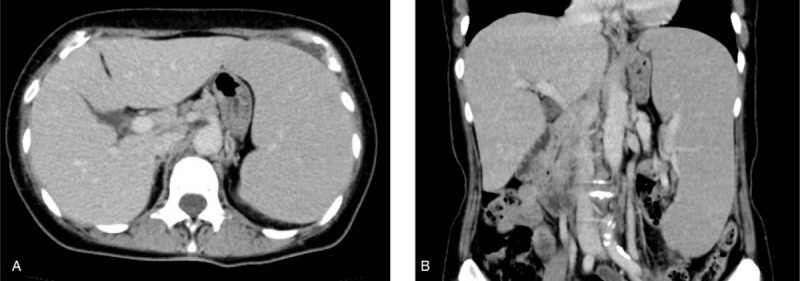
Computed tomography of the abdomen showing splenomegaly. (A) Horizontal axis. (B) Coronal axis.
